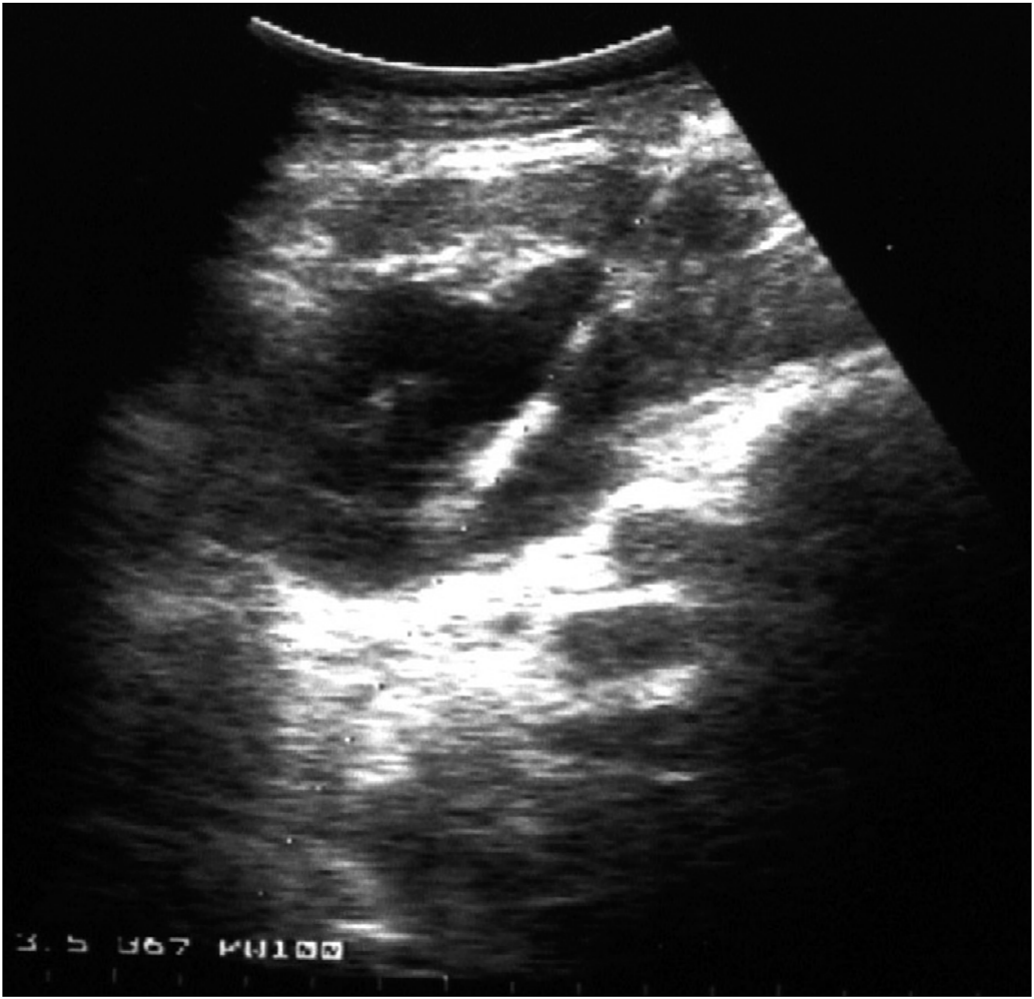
Ultrasound scan of percutaneous drainage of left-sided subphrenic abscess by the stylet-catheter.
